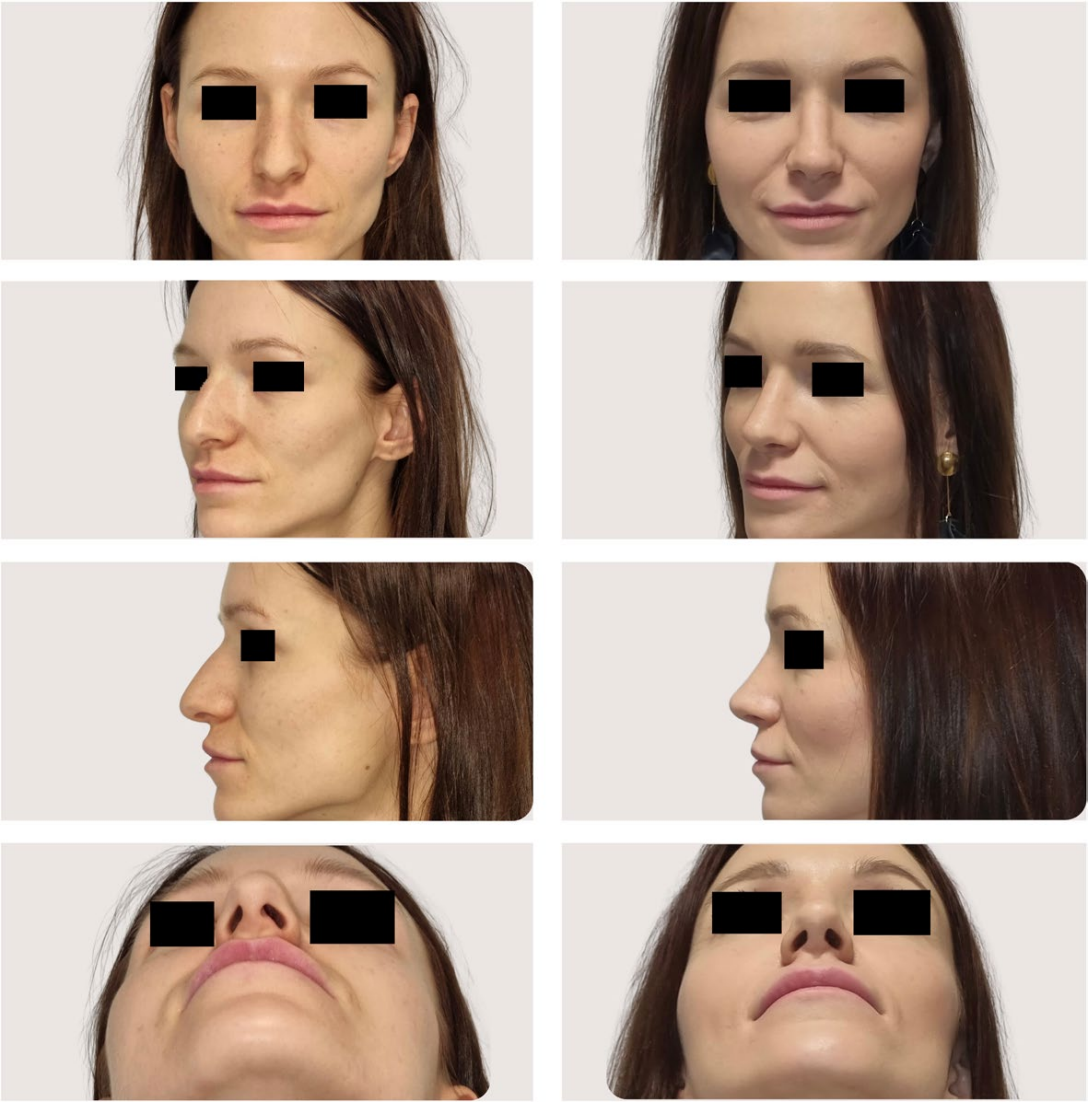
CT-assisted precision rhinoplasty in patient B desiring sensual feminine aesthetic outcome
